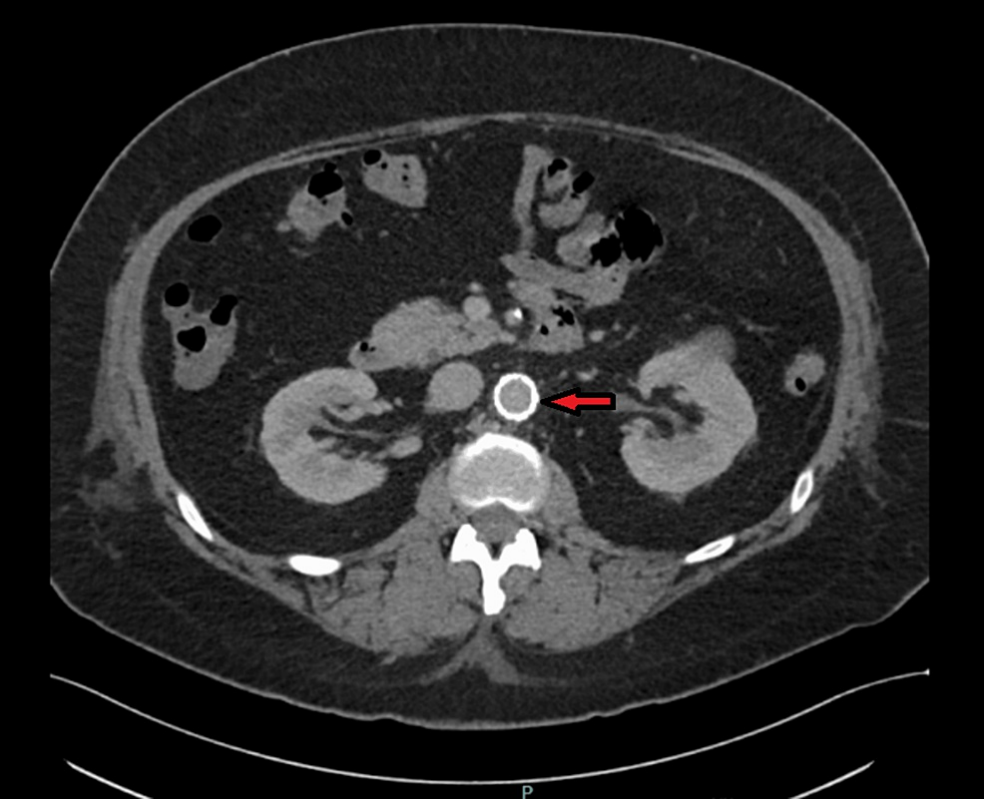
Axial view of CT angiogram of the abdomen post-endovascular repair Axial view of the repeat CT angiogram of the abdomen post-endovascular aortic repair, demonstrating placement of aorto-bi-iliac stent, with similar inflammatory stranding in the region of previously noted penetrating ulcer (arrow).
